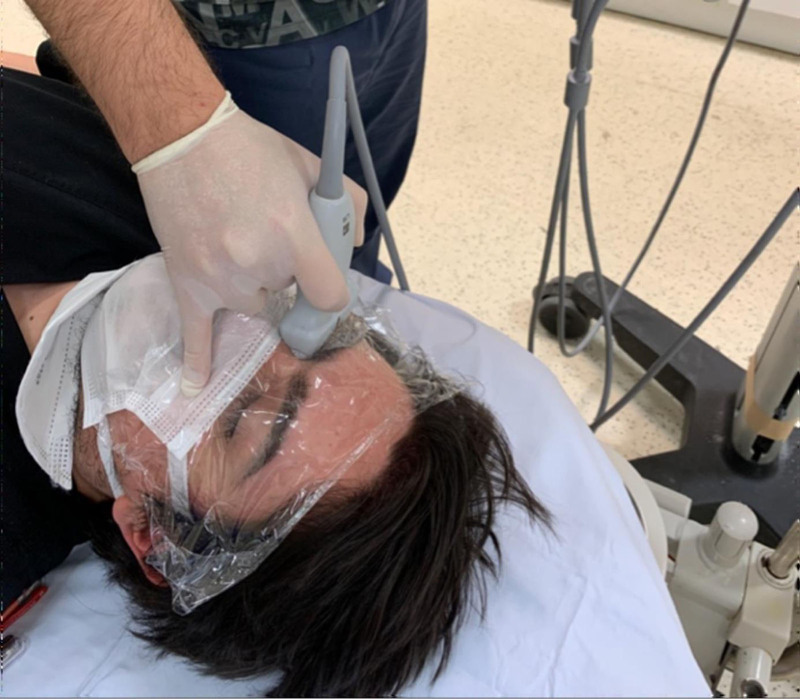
Bedside ultrasonographic measurement of the ONSD in a supine patient. A linear transducer is gently placed over the closed eyelid, with a stretch film barrier and ultrasound gel applied to avoid direct contact. ONSD = optic nerve sheath diameter.
